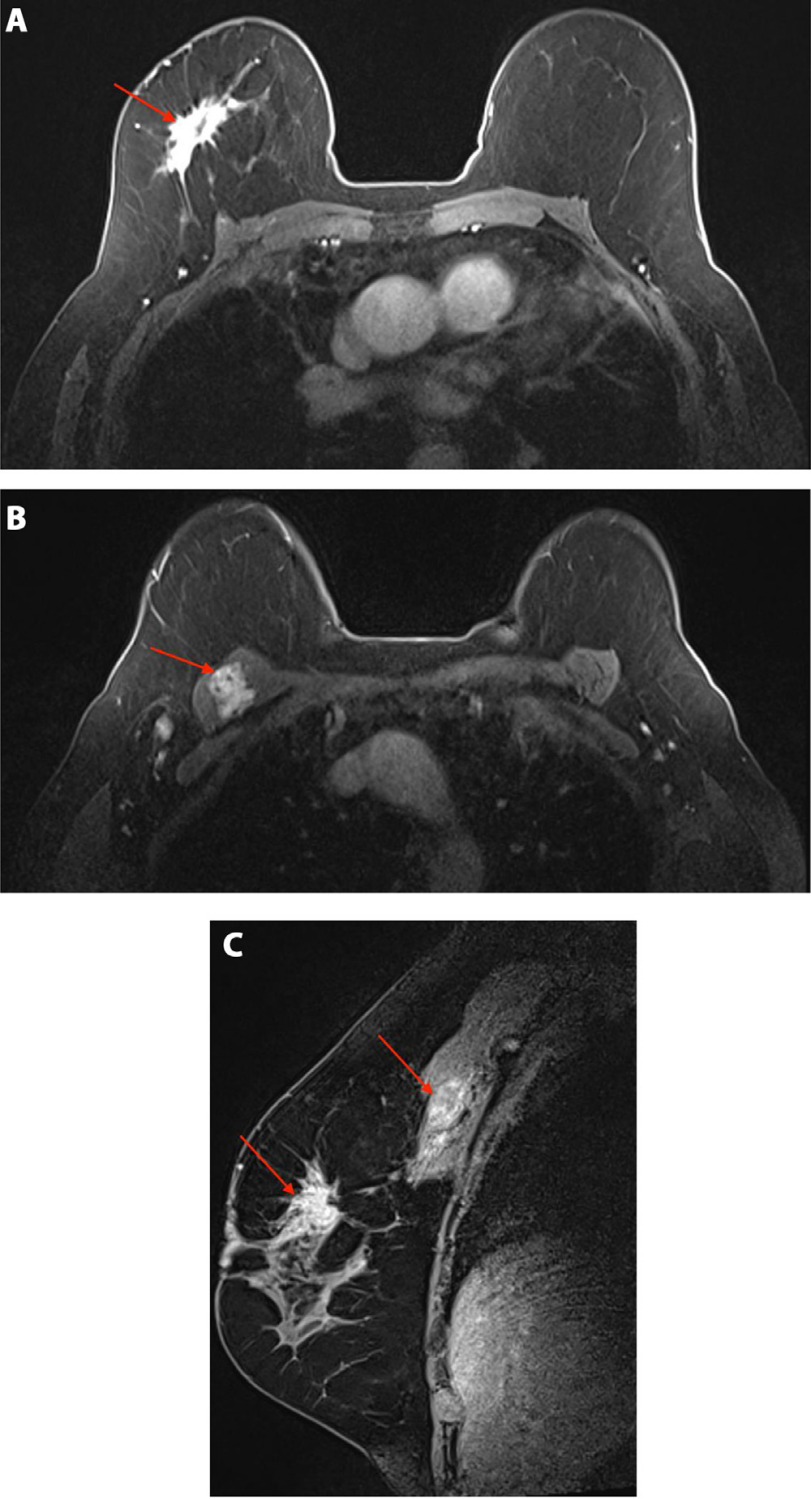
Magnetic resonance imaging of the breasts, contrast enhanced sequences in axial (A, B) and sagittal (C) views. (A) An irregular enhancing mass is visualized at middle depth in the upper outer quadrant of the right breast measuring up to 3.9 cm (arrow). (B) An area of mass enhancement in the right pectoralis major muscle measuring up to 2.1 cm (arrow). (C) Breast and pectoralis major massess (arrows) visualized within the same slice.
